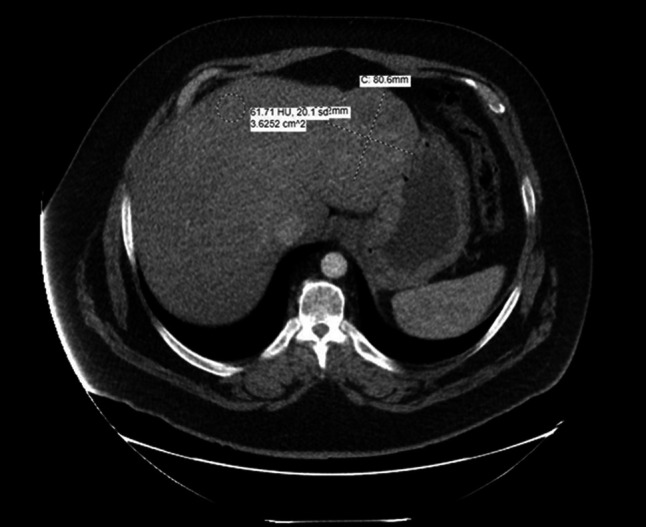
Patient's first computed tomography scan, 7 years prior.
